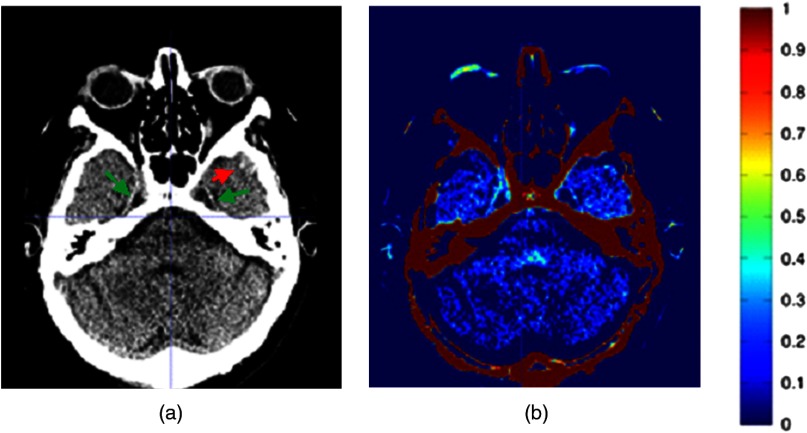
(a) A slice of the original CT image of a TBI patient with bilateral small subdural hemorrhages along Meckel’s cave (green arrows) and an originally missed cerebral hemorrhagic contusion (red arrow). (b) After applying the automatic detection method on the original image, the two subdural hemorrhages were detected, while the hemorrhagic contusion was partially detected. However, the hemorrhagic contusion was not noted in the clinical notes of the patient.
